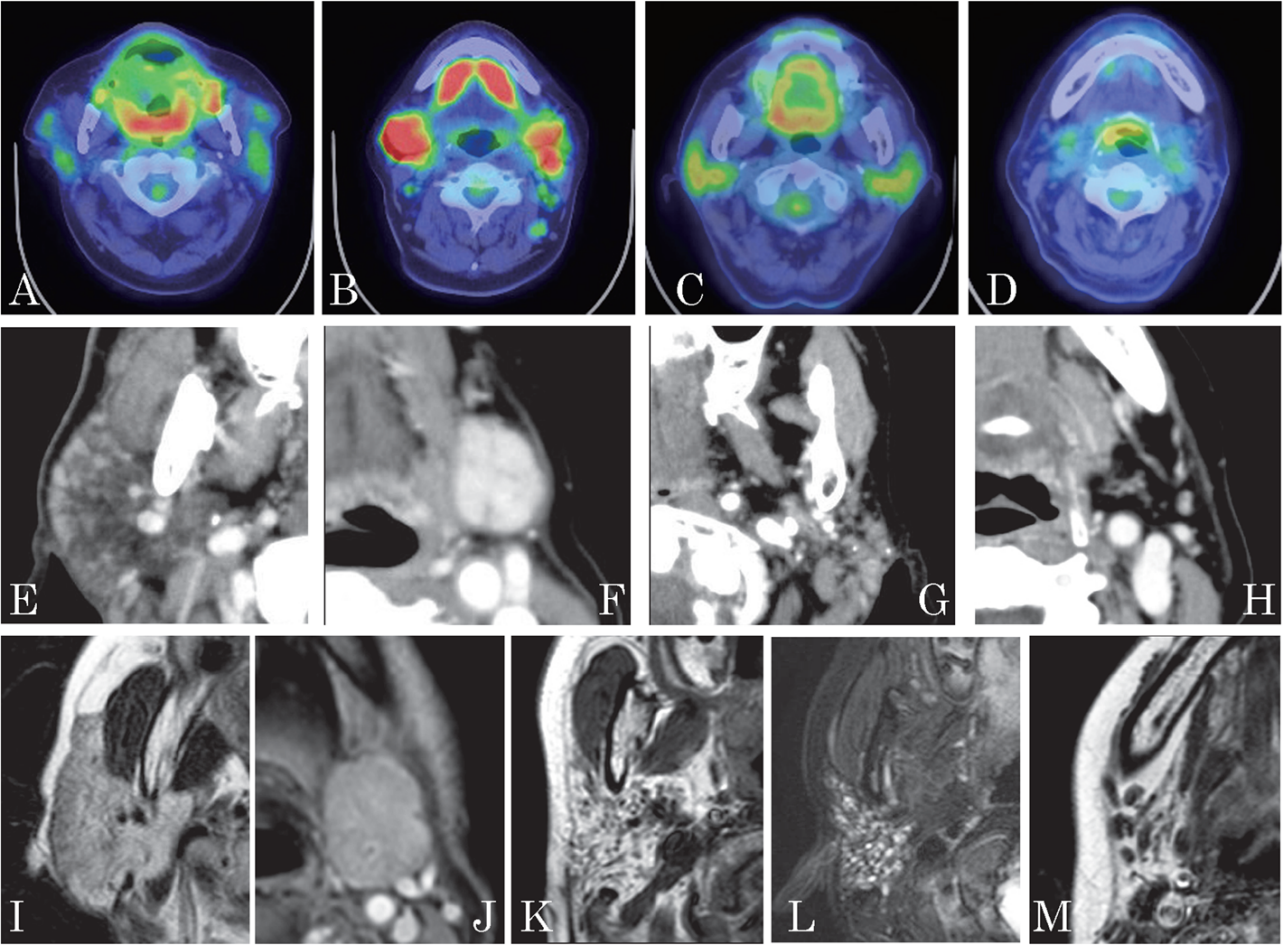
Characteristic findings on FDG-PET/CT, CT and MRI. a-d FDG-PET/CT, e-h CT and i-m MRI. a, e, i Parotid glands of a patient with IgG4-DS. b, f, j Submandibular glands of a patient with IgG4-DS. c, g, k, l Parotid glands of a patient with SS. d, h, m Submandibular glands of a patient with SS. FDG-PET/CT shows abnormal ^18^F-FDG accumulation in the parotid (a, c) and submandibular glands (b). A patient with IgG4-DS showing parotid (e, i) and submandibular gland swelling (f, j), superficial enhancement of the parotid glands (e), and a septum-like structure in the submandibular glands (f). A patient with SS showing atrophic parotid (g) and submandibular glands (h, m), and a salt-and-pepper appearance (g, k, l). It also shows dot-like calcifications on CT (g), and small multiple cystic areas on T2-weighted images (l). *FDG-PET/CT* 2-[^18^F]-fluoro-2-deoxy-D-glucose positron emission tomography/computed tomography, *CT* computed tomography, *IgG4-DS* IgG4-related dacryoadenitis and sialadenitis, *MRI* magnetic resonance imaging, *SS* Sjögren’s syndrome
